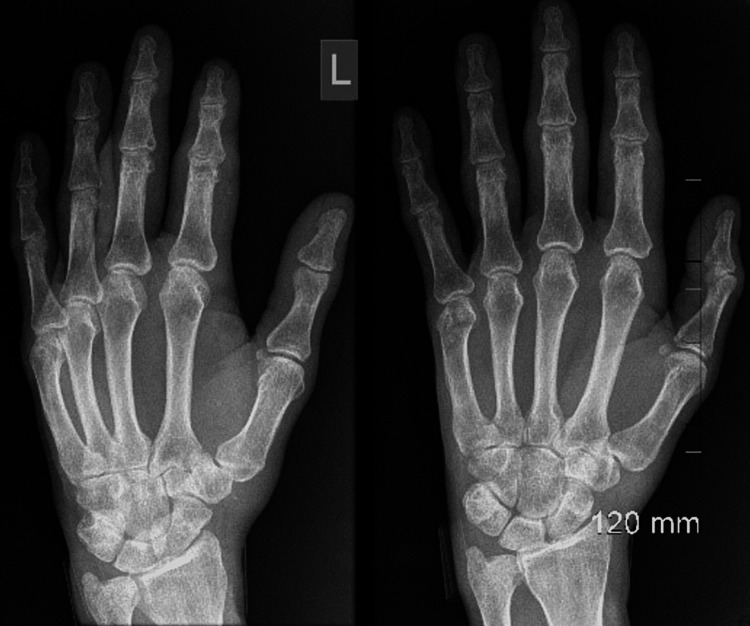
Left hand X-ray during rheumatoid flare-up
